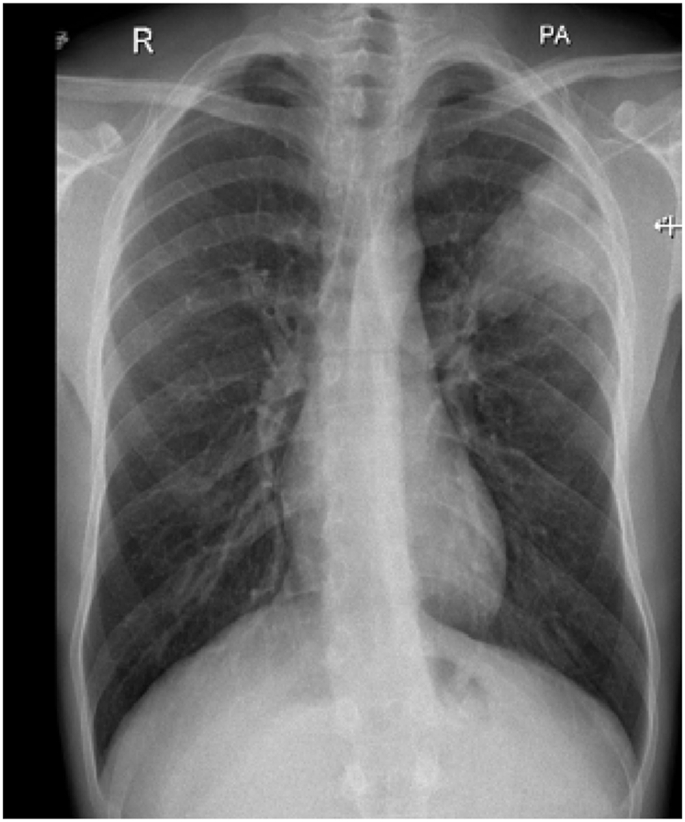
Chest x-ray PA, April 2020: showing left upper lobe mass-like opacity.
